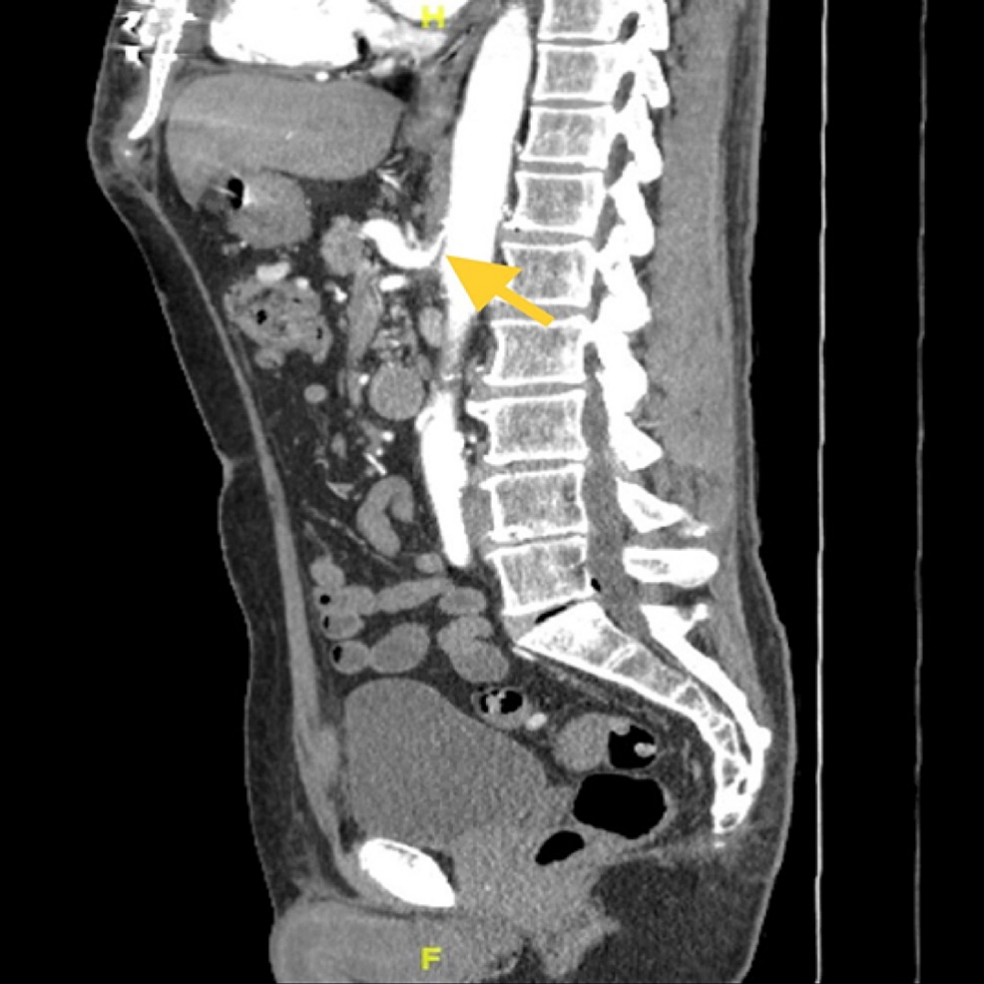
Sagittal view computed tomography angiography (CTA) of the abdomen and pelvis with a yellow arrow showing moderate median arcuate ligament compression of the celiac artery resulting in moderate ostial stenosis and post-stenotic dilatation
